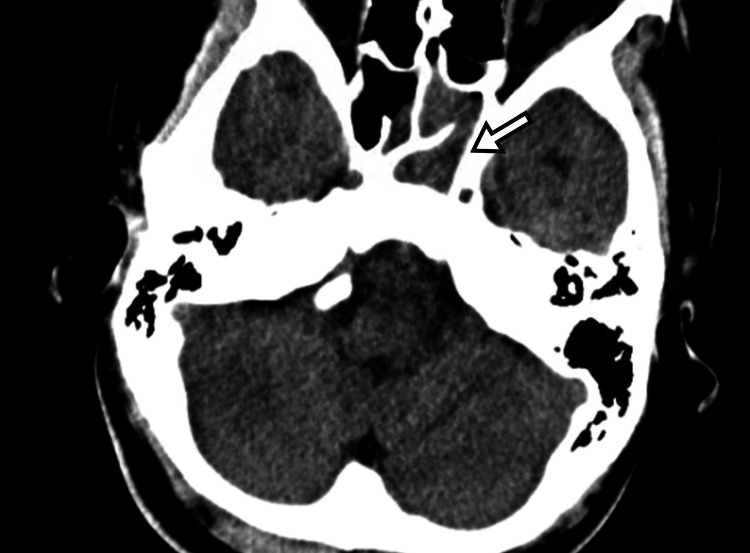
Head computed tomography (CT) on the day of presentation demonstrated fluid retention in the left sphenoid sinus (arrow).
